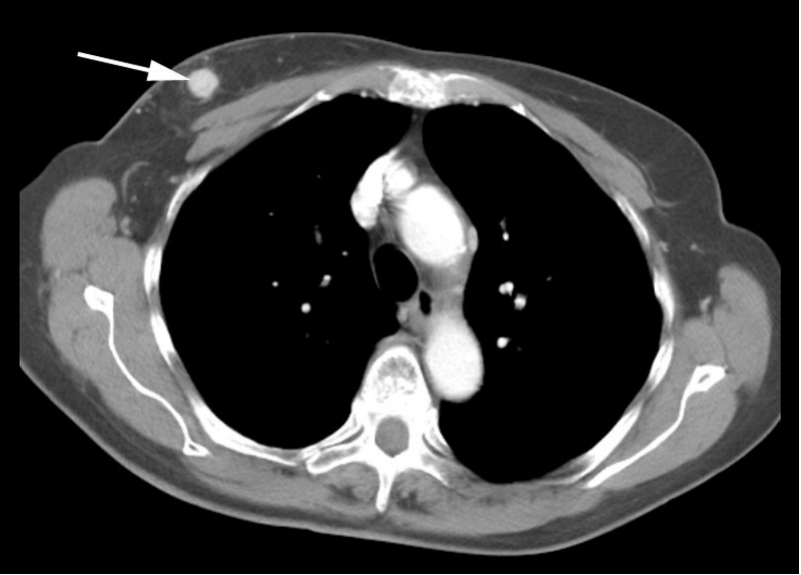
Computed tomography showing well-defined, round mass in the right breast (arrow).
